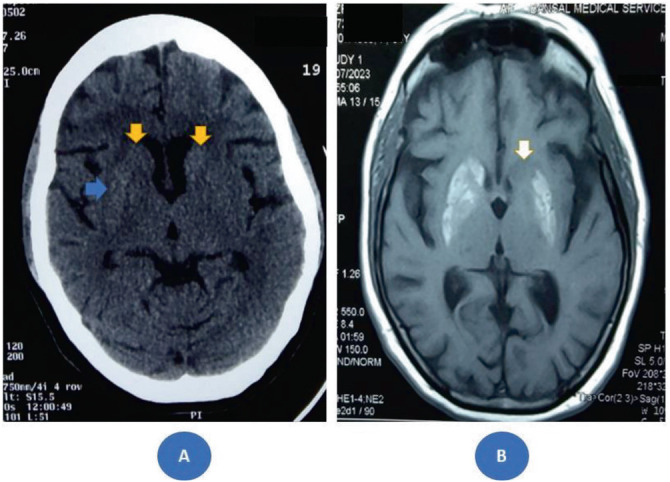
(A) Non-contrast computed tomography scan revealing right putaminal hyperdensity (blue arrow) and subtle bilateral hyperdensity in the head of the caudate nucleus (yellow arrow) at the time of initial presentation. (B) Magnetic resonance imaging displaying a sharply demarcated T1 hyperintense signal in the right striatum, right lentiform nucleus, left putamen, and left lentiform nucleus, with a normal appearance of the left caudate head (white arrow).
